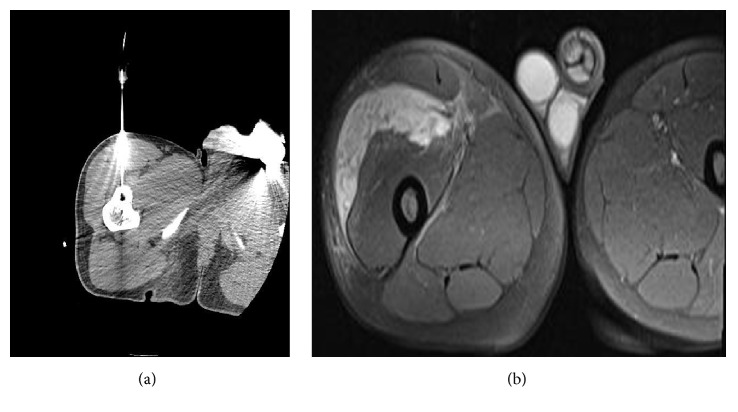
(a) CT image of the lesion located in the proximal femur during the procedure. (b) Hematoma on T2 MRI taken after the procedure.
